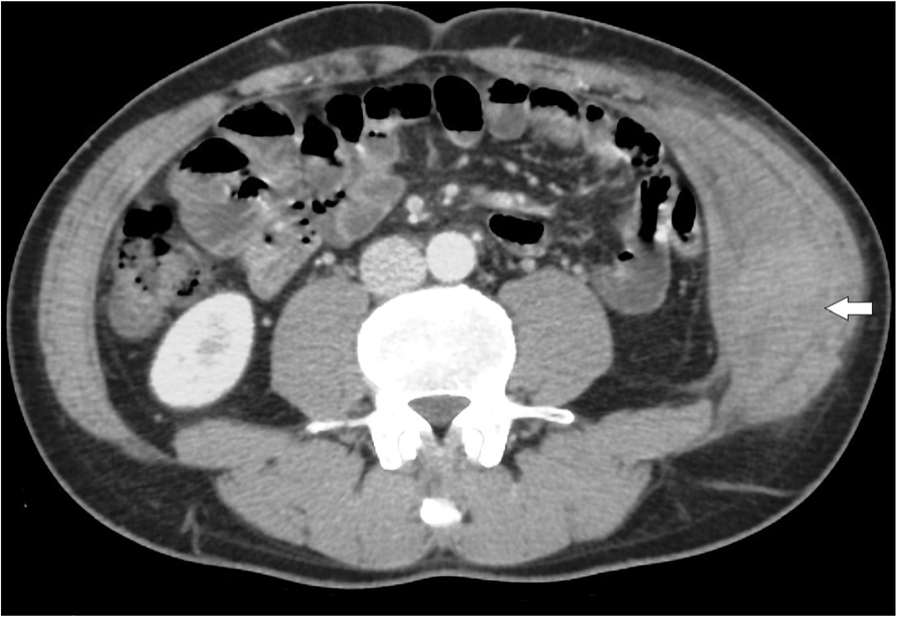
Contrast-enhanced CT. Contrast-enhanced CT did not show extravasation of the contrast material within the mass (white arrow).
